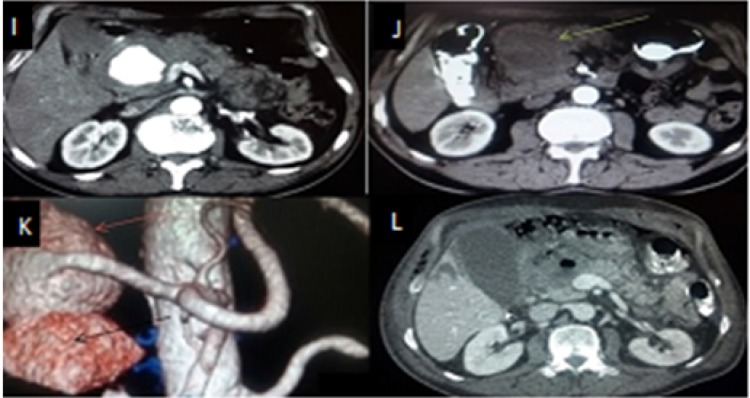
I, J) Axial computed tomography (CT) angiogram showing spontaneous saccular aneurysm in proper hepatic artery with peripheral thrombosis and internal diameter of 44 mm, and evidence of hematoma (white arrow) with compression to second part of duodenum and the head of pancreas. K) Volume-rendered 3D CT angiography image displays aneurysm from common hepatic artery (red arrow) and hematoma (black arrow) below it. L) The patient was referred one week after surgical repairing following abdominal pain. Contrast-enhanced CT (CECT) scan finding showed necrotizing center with severe edema around the head of pancreas with extension to porta-hepatis, peri-portal, and anterior sub-hepatic space suggestive of acute necrotizing pancreatitis
